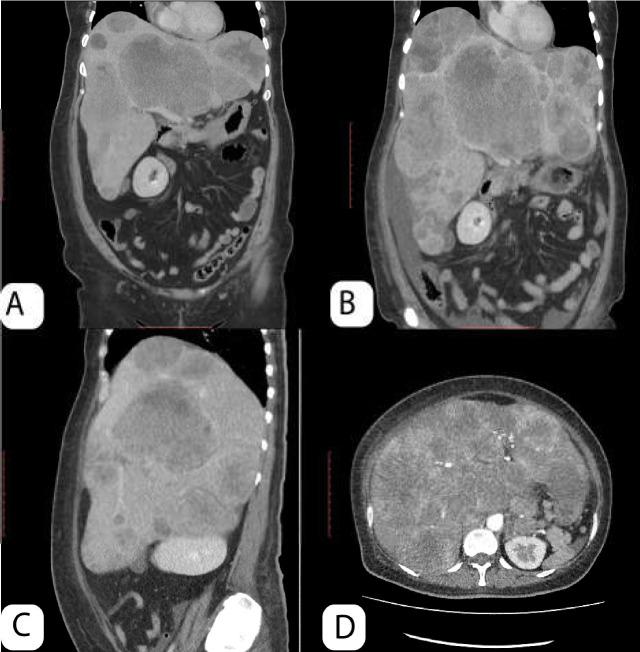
(A) Computed Tomography (CT) at admission shows multiple hypoattenuating liver lesions. (B) CT in coronal plane 2 weeks later with severe hepatomegaly, increasing liver lesions with peripheral rim of enhancement. (C) Sagittal plane demonstrates hepatomegaly fill abdominal cavity and compromises right kidney. (D) Contrast-enhanced computed tomography in arterial phase resembling hepatocellular carcinoma pattern
